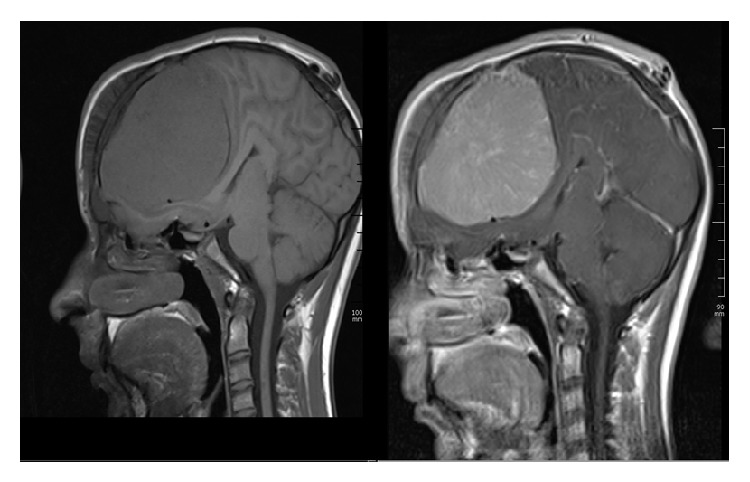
Magnetic resonance imaging. Contrast-enhanced sagittal T1-weighted MRI scan shows an extra-axial frontal tumoral lesion with intense enhancement.
